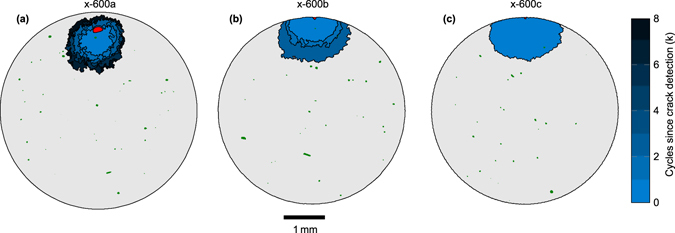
Fatigue crack initiation detected by CT. - Fatigue cracks (blue), the pore that initiated the crack (red), and all other pores (green) within a 1 mm thick slice centred around the plane of the crack, detected by CT after (a) 70 k, (b) 100 k and (c) 120 k cycles. In (a) and (b) multiple crack tidemarks show the detected crack size every 1 k cycles following first detection of the crack.
